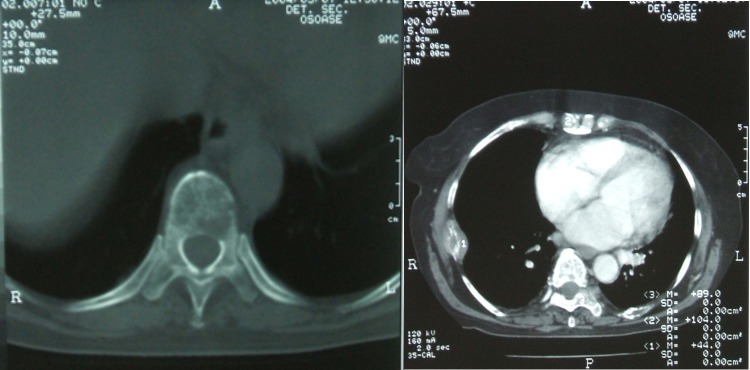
Computed tomography exam showing osteolytic lesions with broken cortical, at the level of the thoracic vertebral bodies, the right ribs and the sternum
